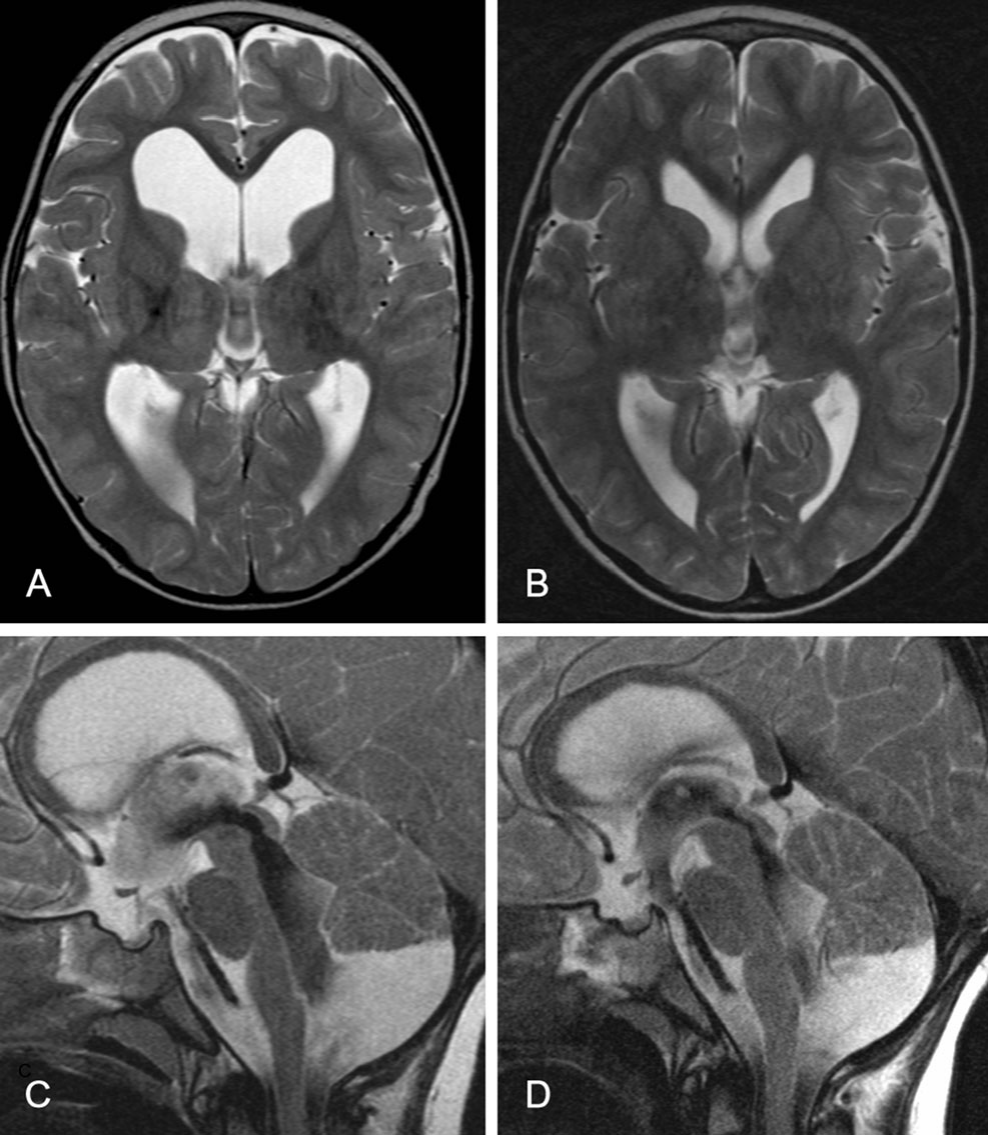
Preoperative axial (a) and sagittal (c) T2-weighted MR images of a child with Blake’s pouch cyst. Axial (b) and sagittal (d) T2-weighted MR images after ETV
